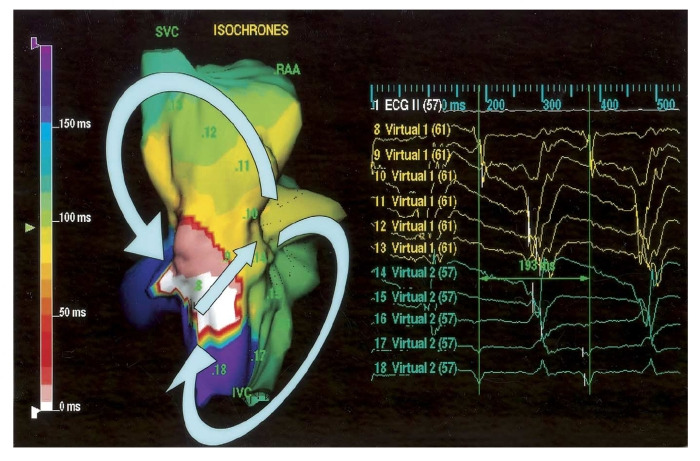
Isopotential map showing type I figure of eight reentry in the right posterior oblique view. The activation wave front propagated through the conduction gap in the crista terminalis (CT) and separated into two wave fronts. The counterclockwise wave front (virtual 8 to 13) rotate around the superior vena cava (SVC) and upper CT, and the clockwise wave front (virtual 14 to 18) rotate around the inferior vena cava (IVC) and lower CT. The virtual elecetrogram 9 at the CT gap showed low amplitude potential between double potentials, representing slow conduction (Reproduced from Tai CT, Liu TY, Lee PC, Lin YJ, Chang MS, Chen SA. Noncontact mapping to guide radiofrequency ablation of atypical right atrial flutter. J Am Coll Cardiol 2004;44:1080-10866, with permission from the American College of Cardiology Foundation.) RAA = right atrial appendage.
